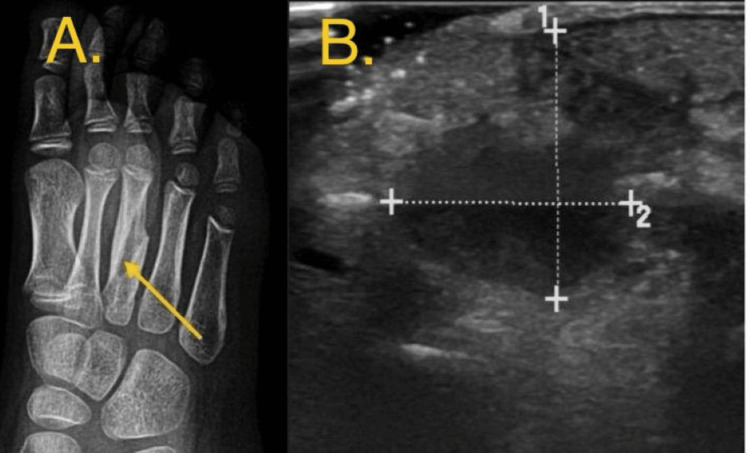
A: Pre-operative oblique radiograph of the foot demonstrating chronic osteolysis, tunneling & relative sclerosis of the third metatarsal shaft. B: Pre-operative ultrasound image showing an irregular hypoechoic collection showing internal echoes within, yet no sizable sonopaque foreign bodies could be appreciated
